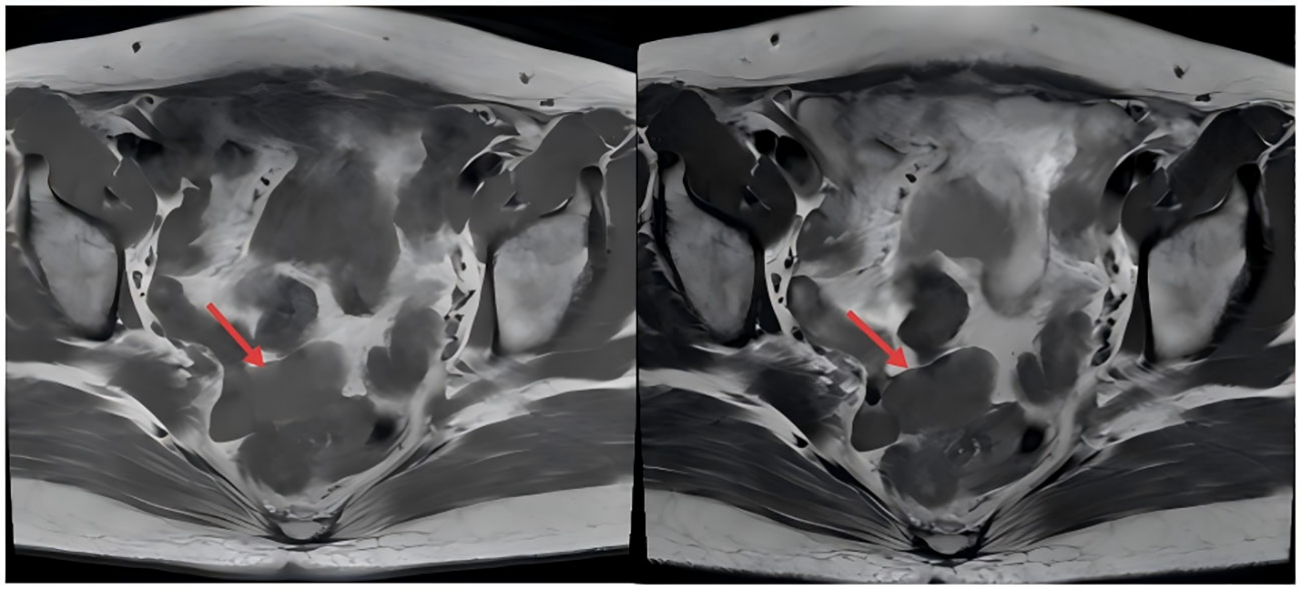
Magnetic Resonance Imaging (MRI) T2-weighted (2023). Red arrows indicate the nodular lesions in the rectouterine pouch.
